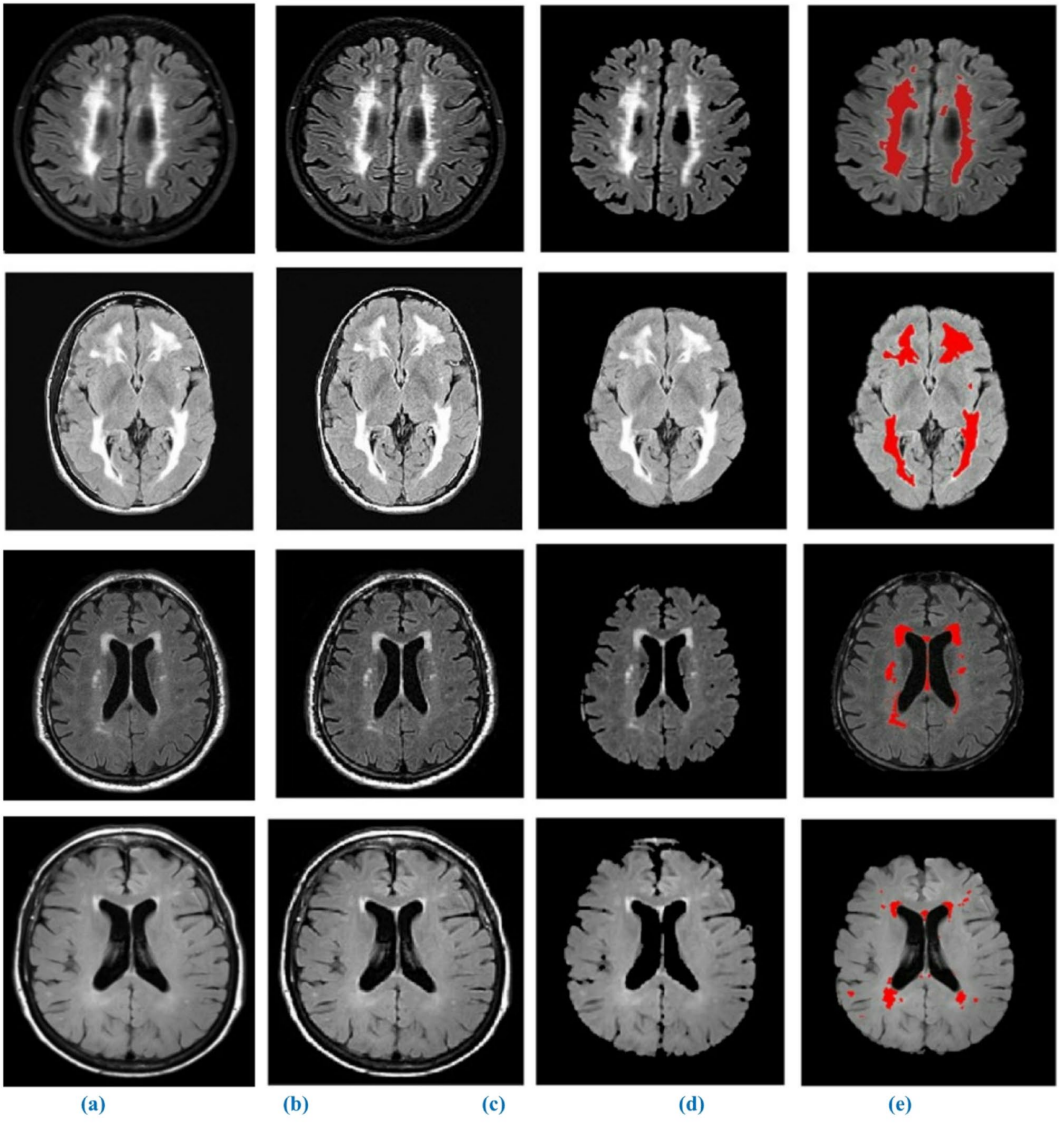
Segmentation of WMH on high-resolution FLAIR MRI images. (a) original image, (b) enhanced image (c) skull stripping, (d) binary mask image, (e) Segmented output.
